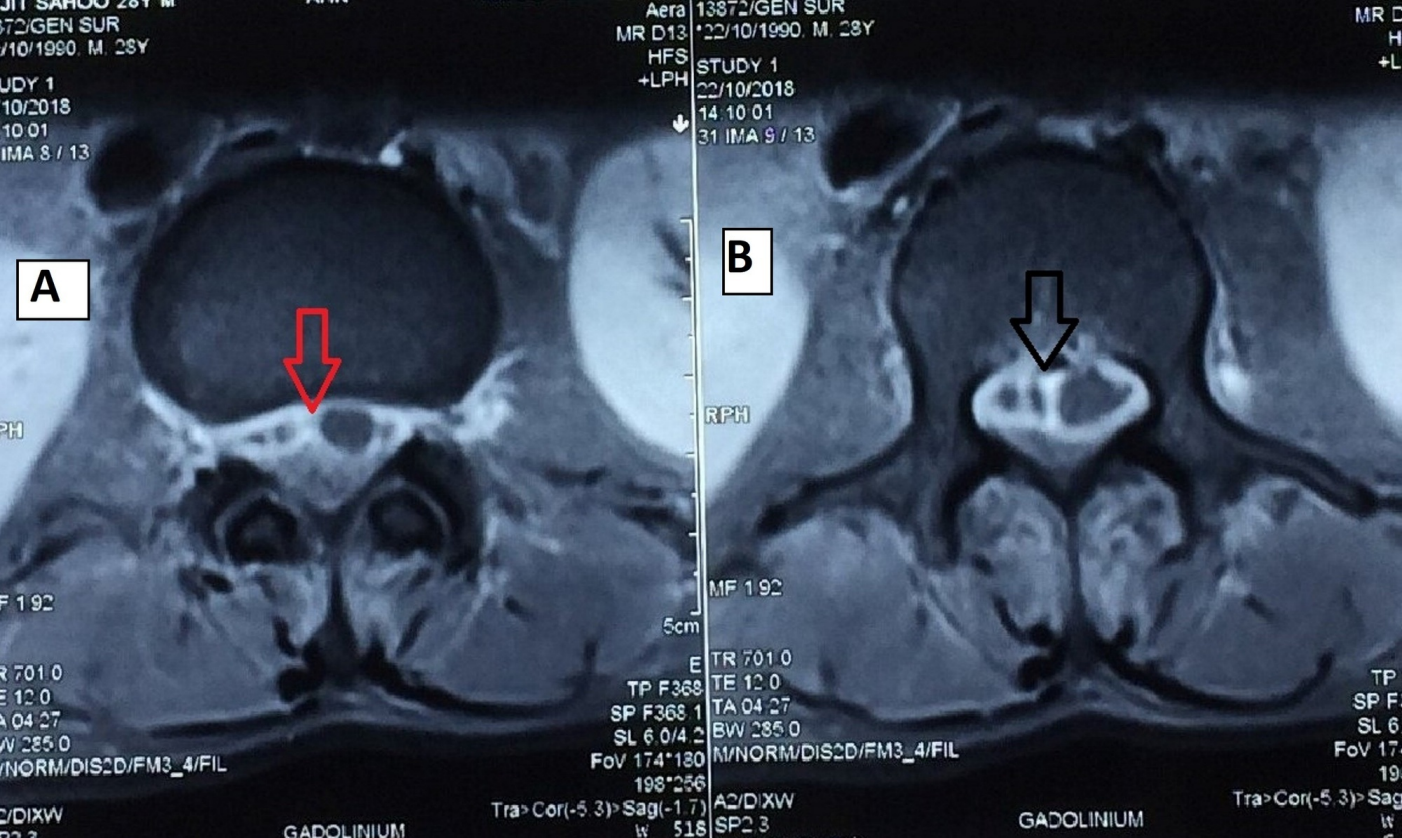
A and B: MRI (axial section) showing a lobulated abscess in the epidural space (arrow mark) MRI: magnetic resonance imaging
